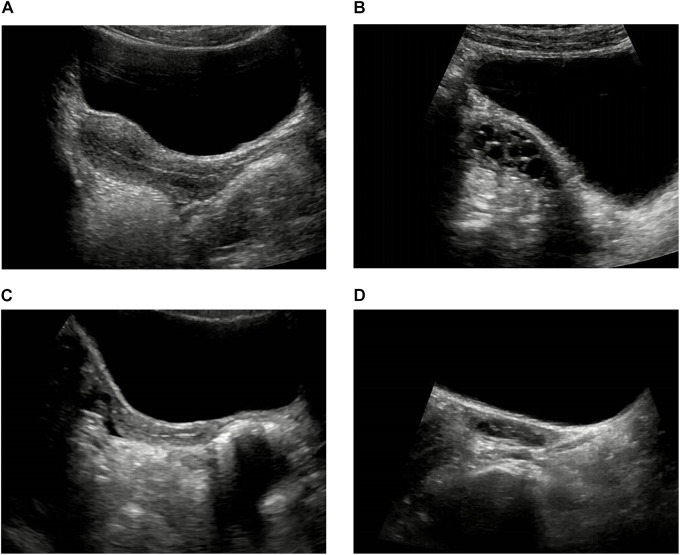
Typical sonograms of the uterus and ovaries of a ICPP patient before and after GnRHa therapy. (A,B) Before GnRHa therapy: the length, anteroposterior diameter, transverse diameter, volume of the uterine body and the ovary, uterine body-cervix ratio were all increased. There were three increased follicles in the ovary, and the maximum diameter of follicle was 8 mm. (C,D) After GnRHa therapy: the length, anteroposterior diameter, transverse diameter, volume of the uterine body and the ovary, uterine body-cervix ratio were all decreased. There was no increased follicle in the ovary.
